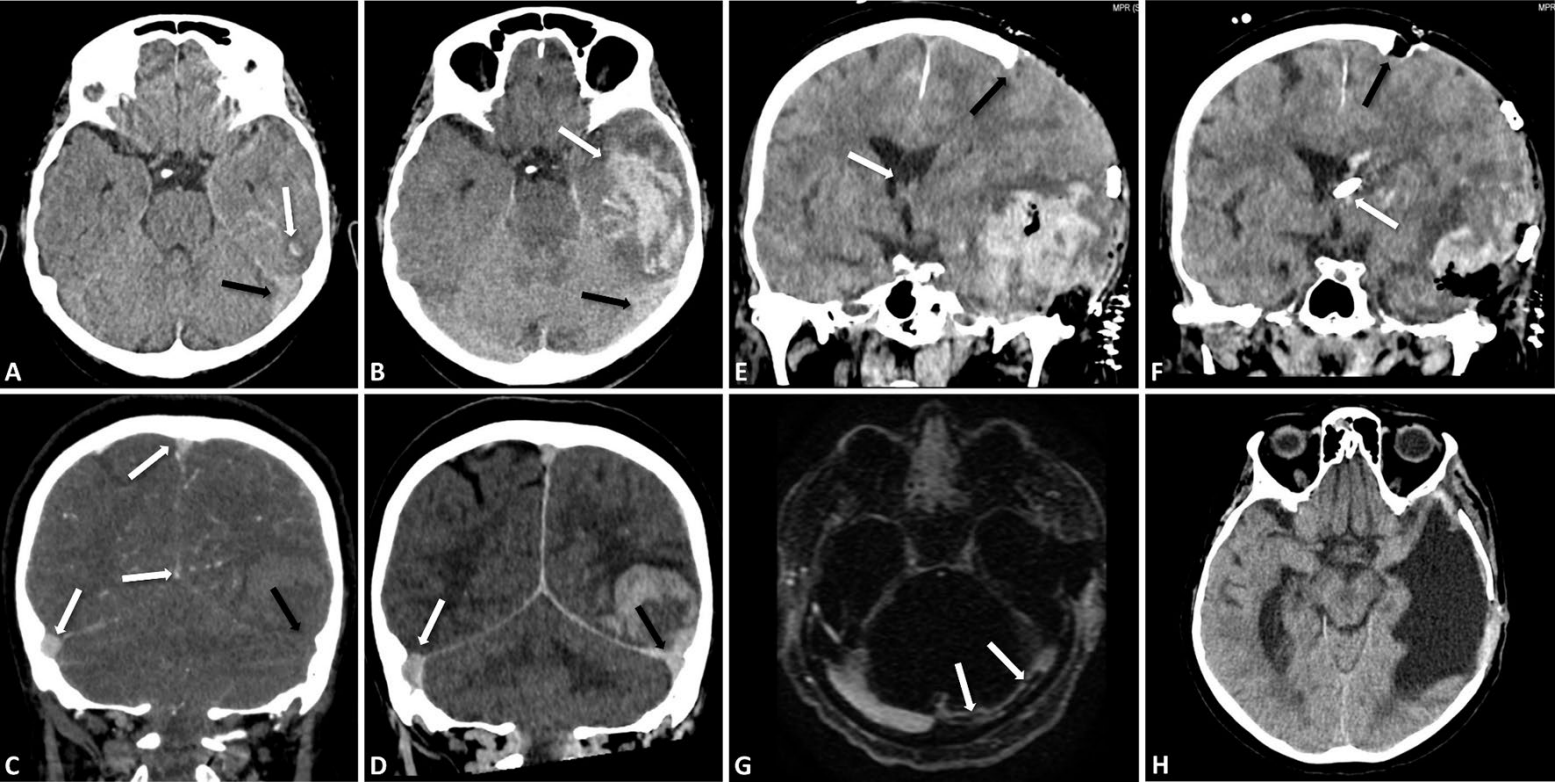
Representative cranial CT (A–F, H) and MRI (G) images throughout the therapeutic course. A Axial slice of the initial cranial CT on the day of admission, with small intracerebral hemorrhage (ICH, white arrow) and mild adjacent subarachnoid hemorrhage (SAH) in the left temporal lobe, as well as discrete “hyperdense triangle sign” of the left transverse sinus (black arrow), suggestive for sinus thrombosis. B Corresponding axial slice of follow-up CT 3 h later with severe progression of left temporal ICH (white arrow) with mass effect, and SAH, and persistent discrete hyperdensity of the left transverse sinus (black arrow), as well as discrete adjacent subdural hemorrhage (SDH). C Coronal slice of the CT-angiography in an arterial phase, acquired immediately after B no signs of aneurysm, arteriovenous malformation or dural arteriovenous fistula. Superior and inferior sagittal sinus, as well as right transverse sinus are already contrasted (white arrows), whereas contrast is missing in the left transverse sinus (black arrow) in the arterial phase, again, suspicious for left-sided sinus thrombosis. In the post-venous phase 30 min later, D there is symmetric contrast enhancement of the somewhat hypoplastic and non-dilated left transverse and sigmoid sinus (black arrow) when compared to the right side (white arrow), ruling against acute occlusive sinus thrombosis. Left-sided cortical veins were difficult to assess because of mass effect, SAH and SDH, but seemed mainly contrasted. E After acute decompressive craniectomy and hematoma evacuation on the day of admission, coronal reformation of the postoperative CT shows recurrent mass effect of relapsing left temporal ICH and occlusive hydrocephalus with relevant midline shift to the right (white arrow) and compression of the cortex at the edge of the craniectomy (black arrow), indicating insufficient decompression. Corresponding coronal slice F of CT after operative revision on the following day with extended craniectomy (black arrow), repeat hematoma evacuation and implantation of an external ventricular drain in the frontal horn of the left lateral ventricle (white arrow), shows sufficient decompression. An axial reconstruction of a dynamic contrast-enhanced MRI angiography in the venous phase G on day 8 shows non-occlusive thrombosis of the hypoplastic left transverse sinus (white arrows), reaching from the torcula to the jugular foramen. Axial slice of the final CT H after bone flap reinsertion 4 months later with large porencephalic defect of the left temporal lobe after complete resorption of the hemorrhage, and no further complications
